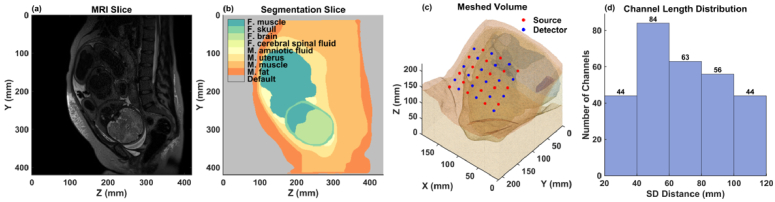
Fetus in Occiput Posterior (OP) position. (a) Sagittal MRI slice. (b) Segmentation of (a). (c) 3D mesh with SD grid on the maternal abdomen. (d) Channel length distribution of the SD grid in (c).
